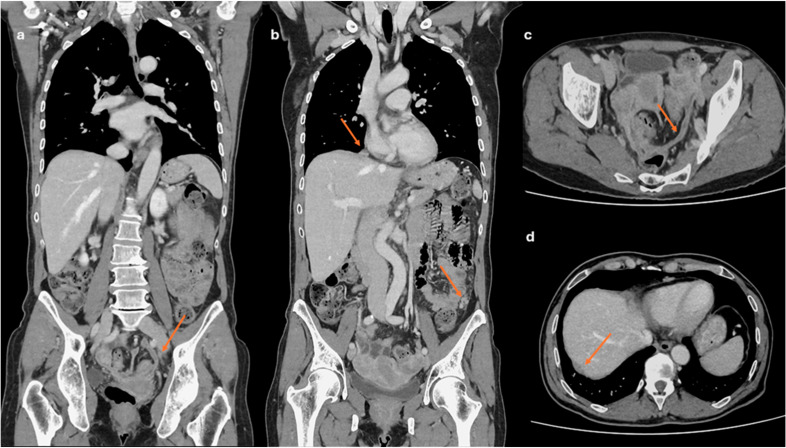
Venous-phase CT staging of ovarian cancer (OC): multiplanar reconstructions (MPR) are mandatory for detecting peritoneal disease (arrow) in the pelvis (a,c), the subphrenic space (d), and along the paracolic gutter ((b), lower arrow). CT is also necessary for detection of suspicious lymph nodes ((b), upper arrow).
